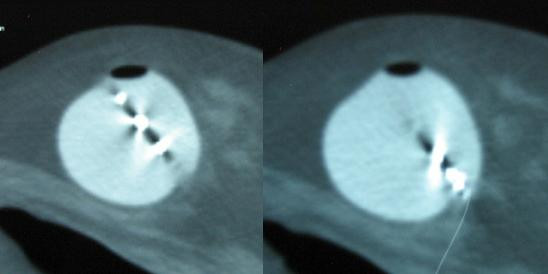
CT scan slices from case 1 showing the asymmetry of the center channel.
